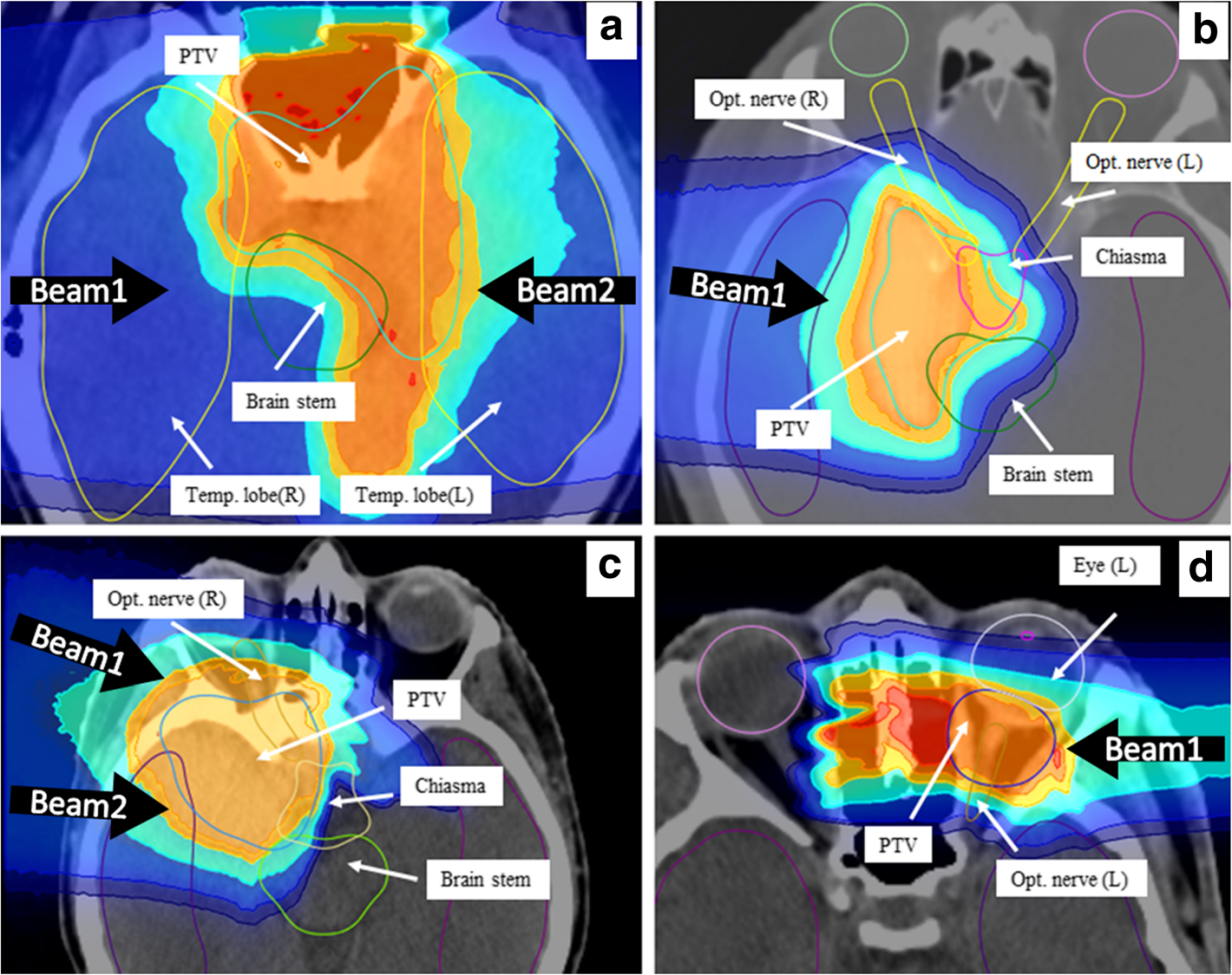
The four panels (a-d) display the complex relation between the contoured PTV and OARs for the four considered patient cases on the X-ray computed tomography axial images (gray scale), with overlaid dose distributions (color wash) for proton plans of fixed RBE calculated with MCTP. Second beam for patient B (panel b) is following the head-feet axis
